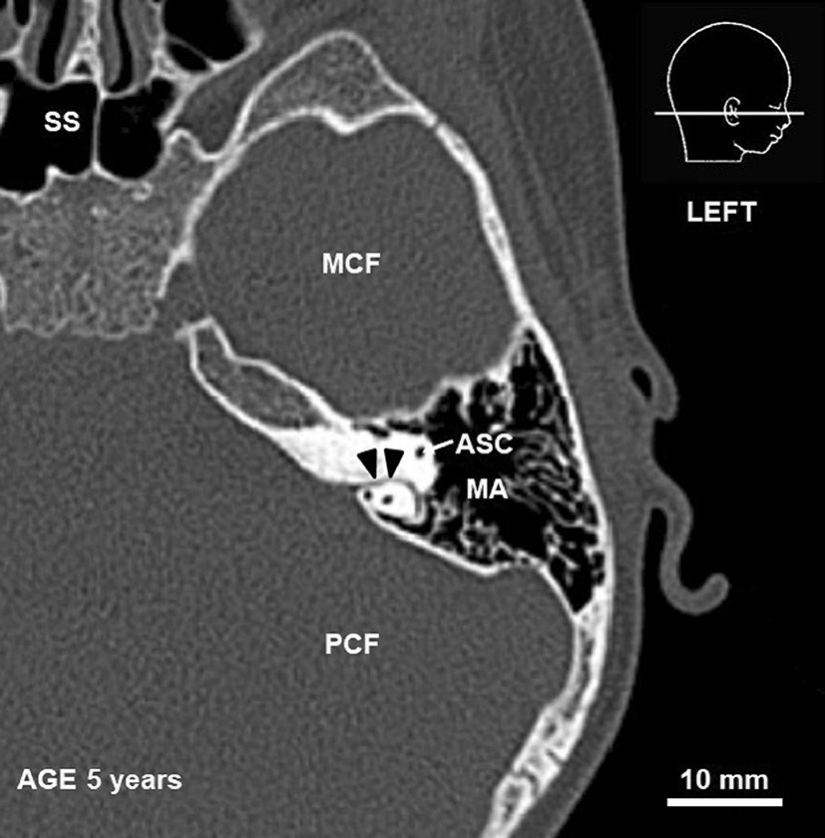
CT scan of a 5-year-old child. Arrowheads indicate subarcuate canaliculus, *ASC* anterior semicircular canal, *MCF* middle cranial fossa, *MA* mastoid antrum, *PCF* posterior cranial fossa, *SS* sphenoid sinus
